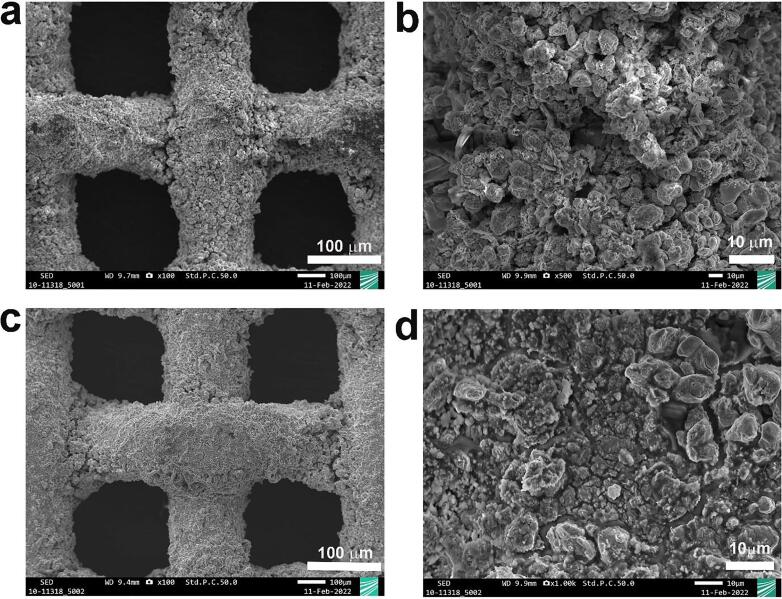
Top-view SEM images (SE mode) of Raney-Ni at different magnifications: a) and b) without ultrasonication (*silent* conditions), c) and d) after ultrasonication for 15 min in 30 wt.-% aqueous KOH solution *T* = 25 °C.
